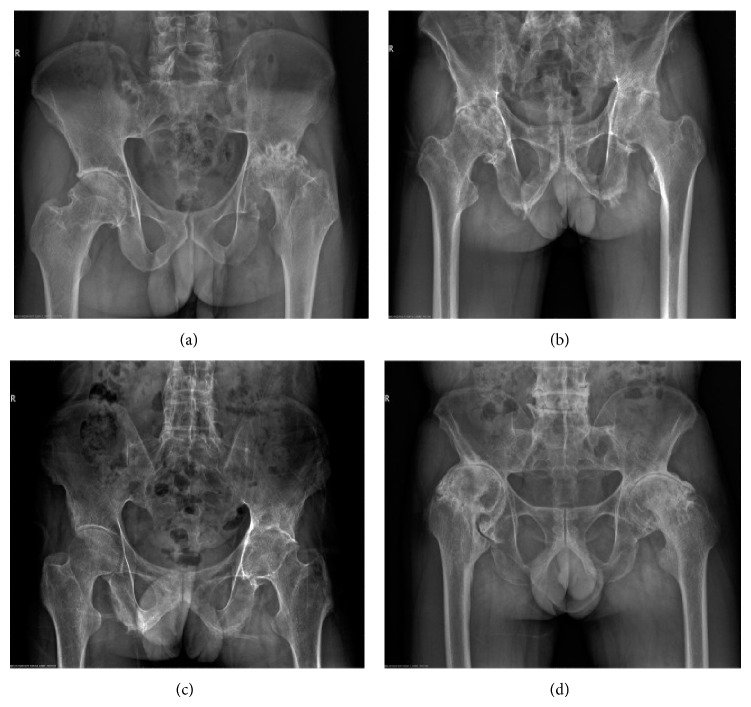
Radiographic findings in AS patients with advanced hip joint disease. (a) Case 3; (b) case 5; (c) case 6; and (d) case 7.
